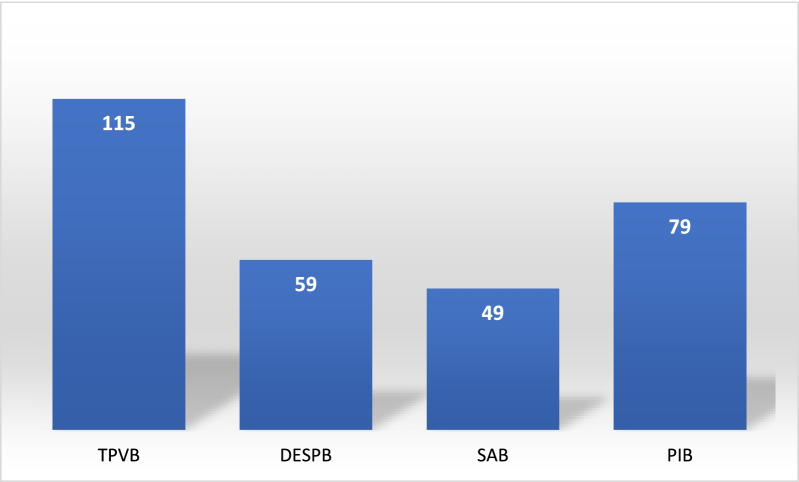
Regional analgesia techniques (numbers of patients). *TPVB: Thoracic paravertebral block, DIESPB: Dual-injection erector spinae plane block*. *SAB: Serratus anterior plane block, PIB: Parasternal intercostal block*
